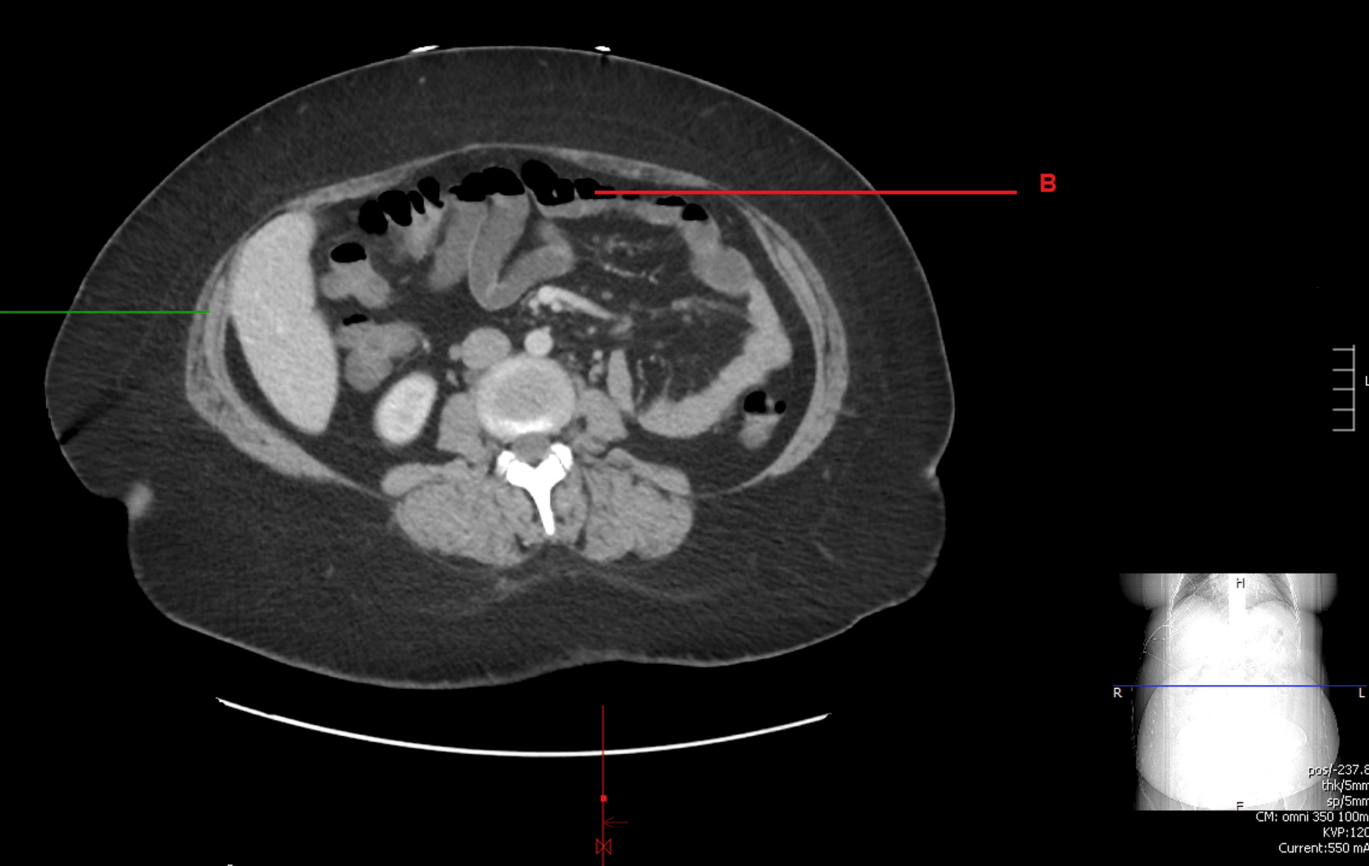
CT abdomen/pelvis with contrast showing fluid-filled bowel loops and colonic fluid (indicated by the red line marking B).
